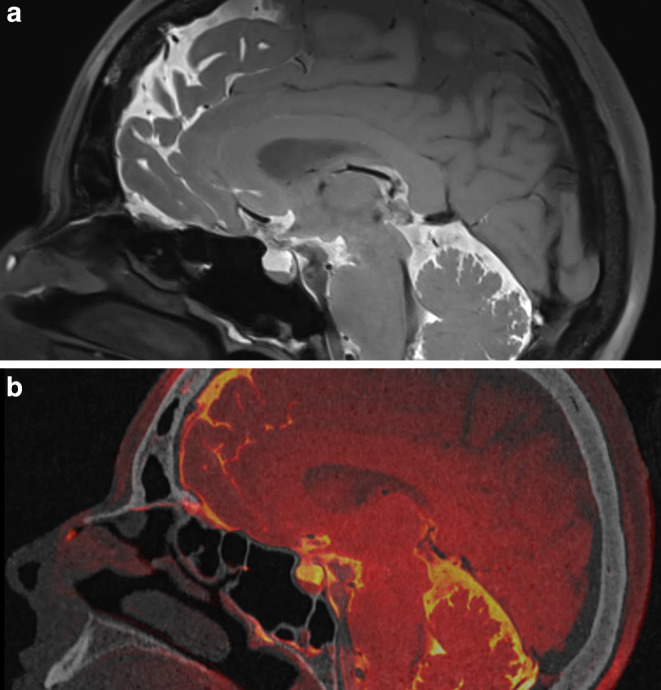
Sagittal section of (a) intrathecal gadolinium enhanced magnetic resonance cisternography (MRC)  and (b) fusion images of MRC and contrast enhanced computed tomography cisternography (CTC) of patient 1. Both images show clear depiction of CSF leak at left cribriform plate anterior to crista galli
